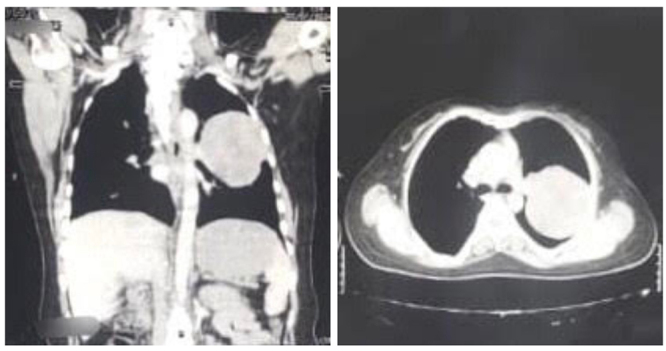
Computed tomographic scan of the chest showing heterogenous soft tissue attenuation mass in the left upper and lower lobe of the lung.
